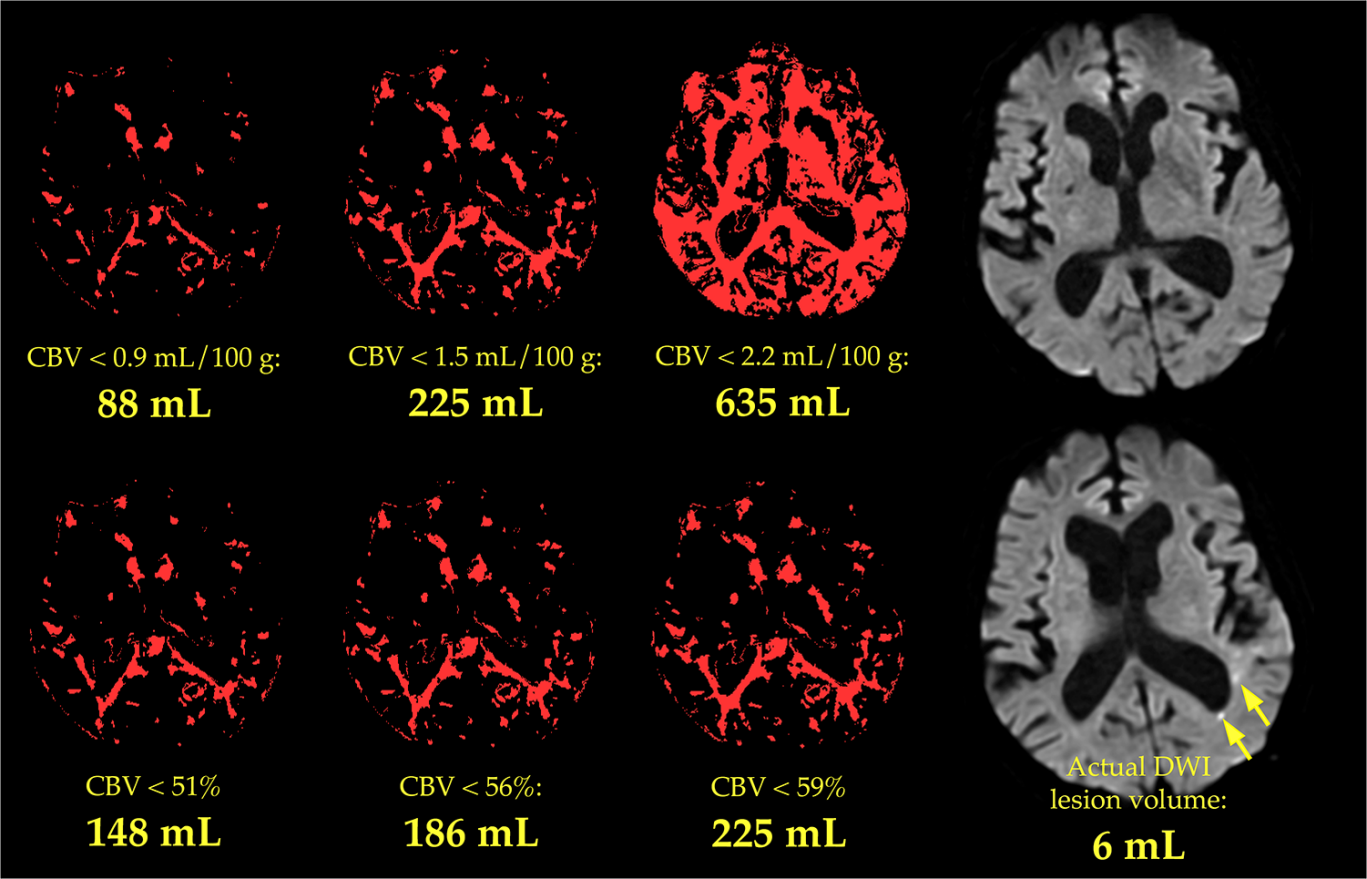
Objective measurement of CBV lesion volume in a second patient. The six smaller images depict low-CBV lesions that were delineated automatically, in a single slice of a second patient’s CBV maps, by rendering in white all brain pixels in which CBV was below each of six different thresholds. Each threshold is listed below its corresponding image, along with the total volume of tissue with CBV below that threshold in all slices. For clarity, the lesions identified by only three of the six absolute thresholds are shown in the top row, and lesions identified by only three of the six relative thresholds are shown in the bottom row. No lesions were seen in a DWI image acquired at approximately the same level (upper right), although DWI images acquired more superiorly showed several small lesions whose total volume was 6 mL (bottom right, arrows).
